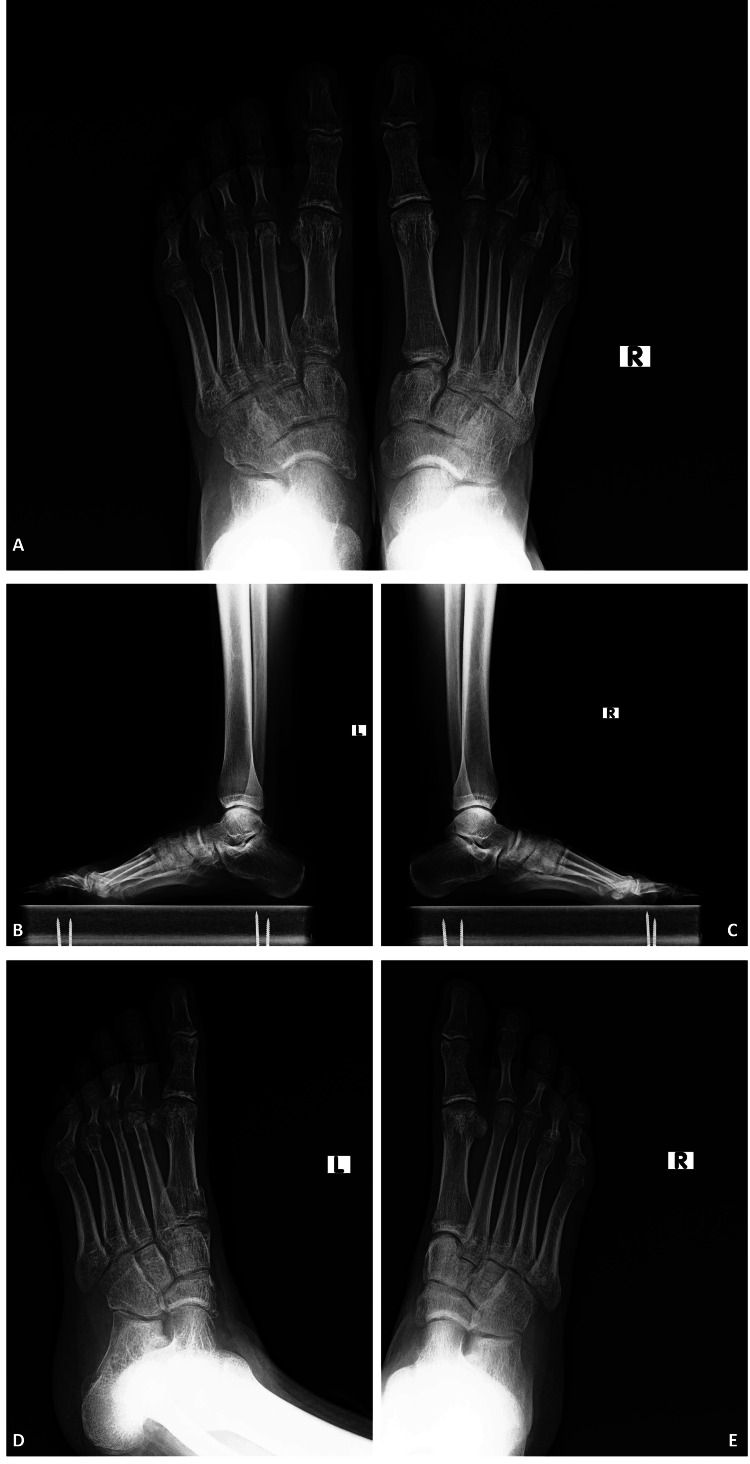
The left and right foot's X-ray images at eight weeks postoperatively A: The left and right foot's anteroposterior X-ray image at eight weeks postoperatively showed the proper alignment of all metatarsals and phalanges. B: The left foot's lateral X-ray image at eight weeks postoperatively showed the proper alignment of all metatarsals and phalanges. C: The right foot's lateral X-ray image at eight weeks postoperatively showed the proper alignment of all metatarsals and phalanges. D: The left foot's oblique X-ray image at eight weeks postoperatively showed the proper alignment of all metatarsals and phalanges. E: The right foot's oblique X-ray image at eight weeks postoperatively showed the proper alignment of all metatarsals and phalanges.
